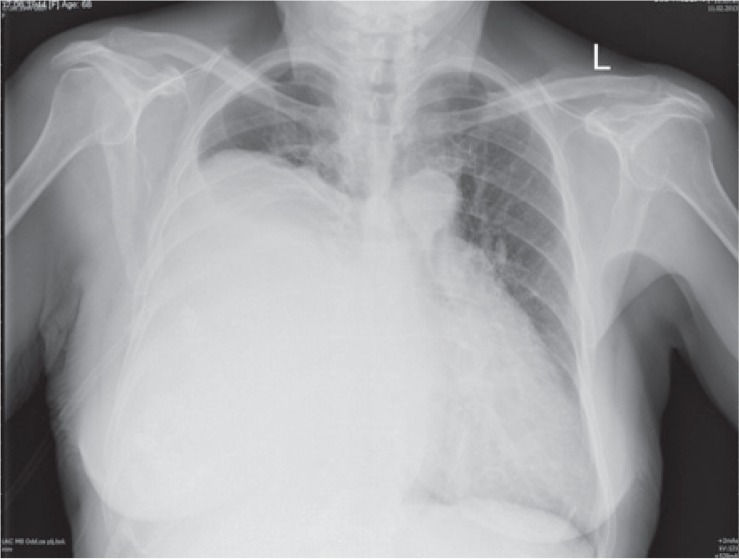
Chest X-ray - a large tumorous process of the right hemithorax with mediastinal shift to the left.
